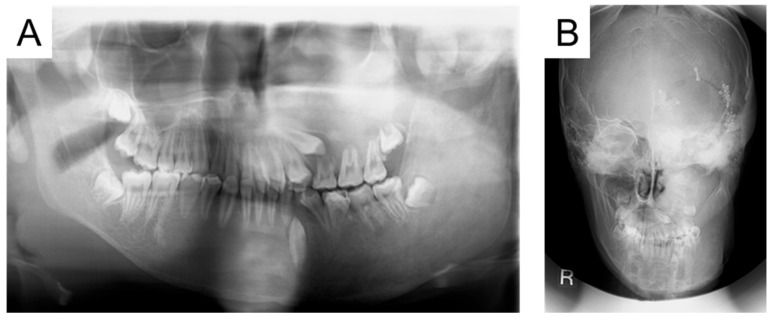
Radiographic examination at the age of 15 years and 6 months ((A): Panoramic photograph, (B): Antero-posterior-view skull).
